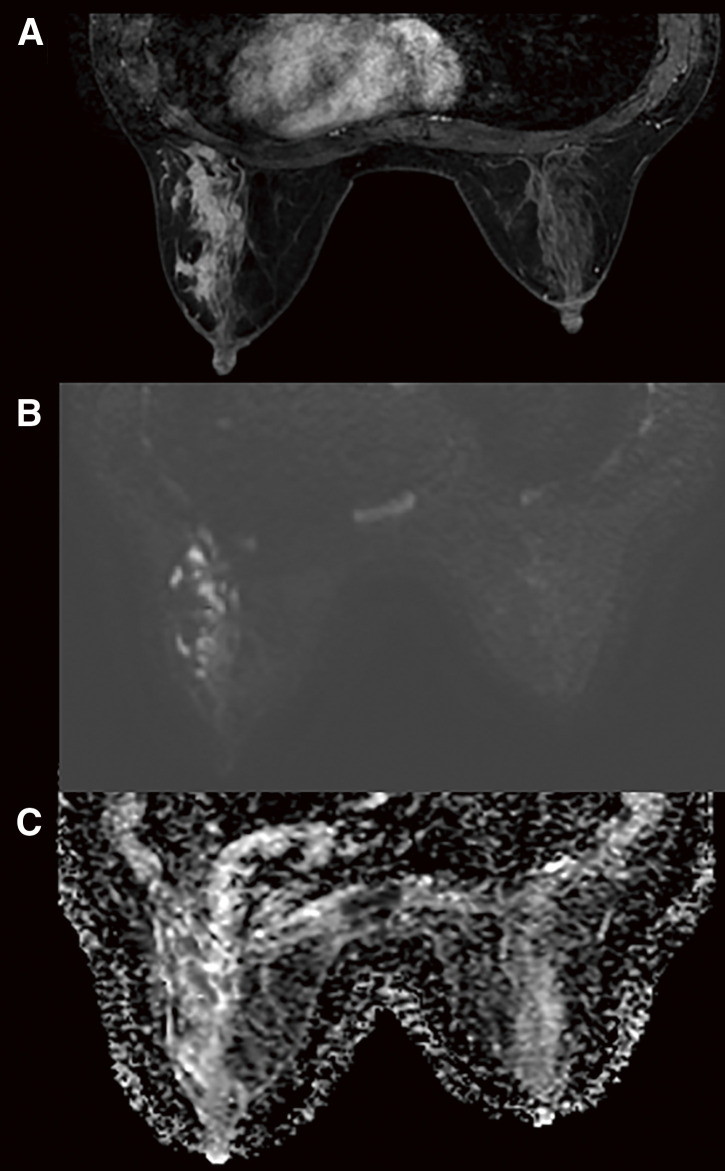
Contrast-enhanced MRI in a case of cystic neutrophilic granulomatous mastitis caused by *Mycobacteroides abscessus*. (A–C) Contrast-enhanced MRI showing a segmental non-enhanced area measuring approximately 36 × 29 × 89 mm. The contrast pattern increased progressively. Diffusion-weighted imaging shows a high signal intensity, and apparent diffusion coefficient mapping shows some low-intensity signals, strongly suspicious for DCIS or invasive carcinoma with a predominant intraductal component. DCIS, ductal carcinoma in situ; MRI, magnetic resonance imaging.
